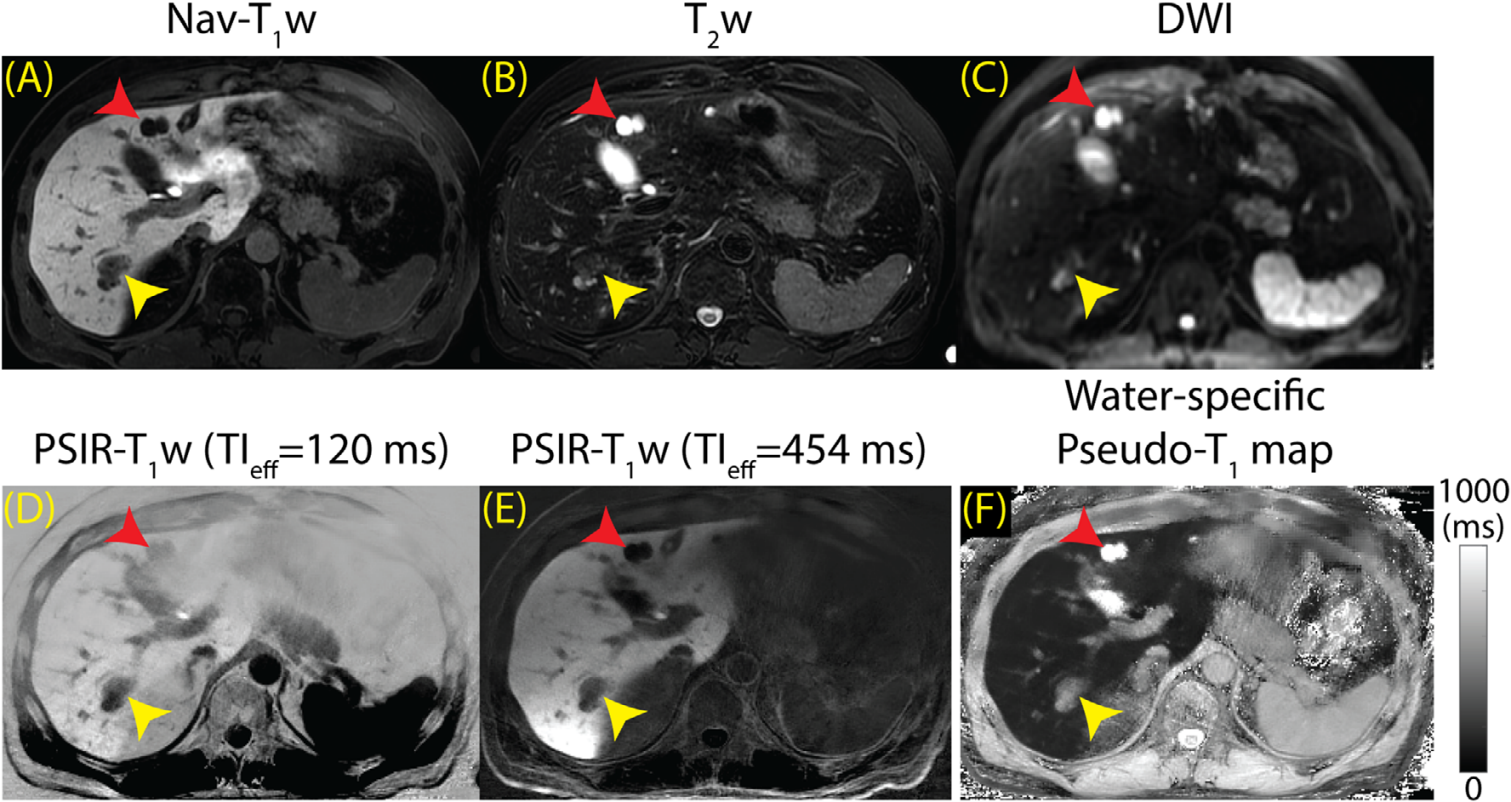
The proposed method improves lesion visibility and characterization by providing multi‐contrast PSIR‐T_1_w images in a single, free‐breathing scan (15 min post‐contrast). Liver metastasis and a simple cyst are shown with yellow and red arrowheads, respectively. (A) Nav‐T_1_w image has superior image quality compared with T_2_w and DWI MRI. (B, C) The simple cyst appears bright in T_2_w and DWI images, whereas metastasis is not visible. (D, E) Lesion visibility was good in PSIR‐T_1_w images acquired at TI_eff_ of 120 and 454 ms. (F) The simple cyst and metastasis had longer pseudo‐T_1_ of 1844 and 221 ms compared to 172 ms of liver parenchyma.
